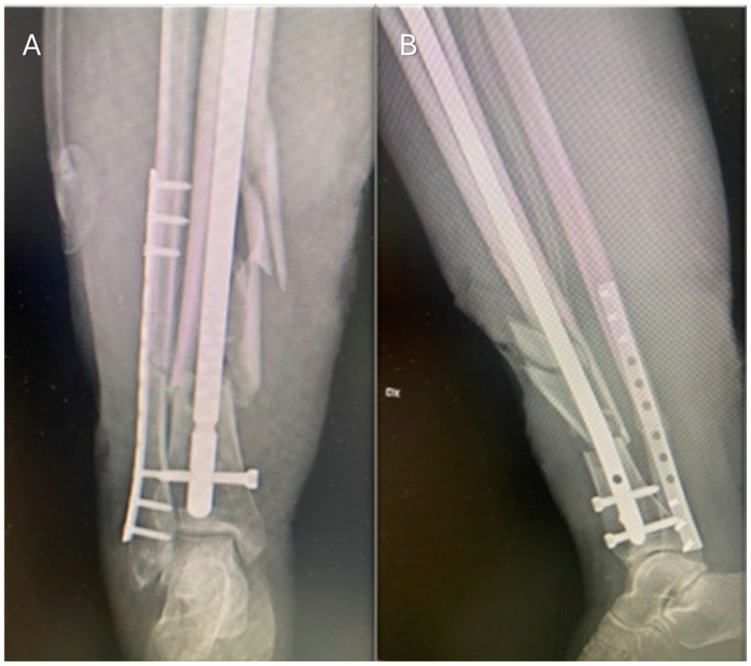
Post-operative X-rays. (A) Anteroposterior view. (B) Lateral view. Fixation of tibia with intramedullary nailing with proximal and distal locking. Stabilization of fibula using plate and screw fixation system.
